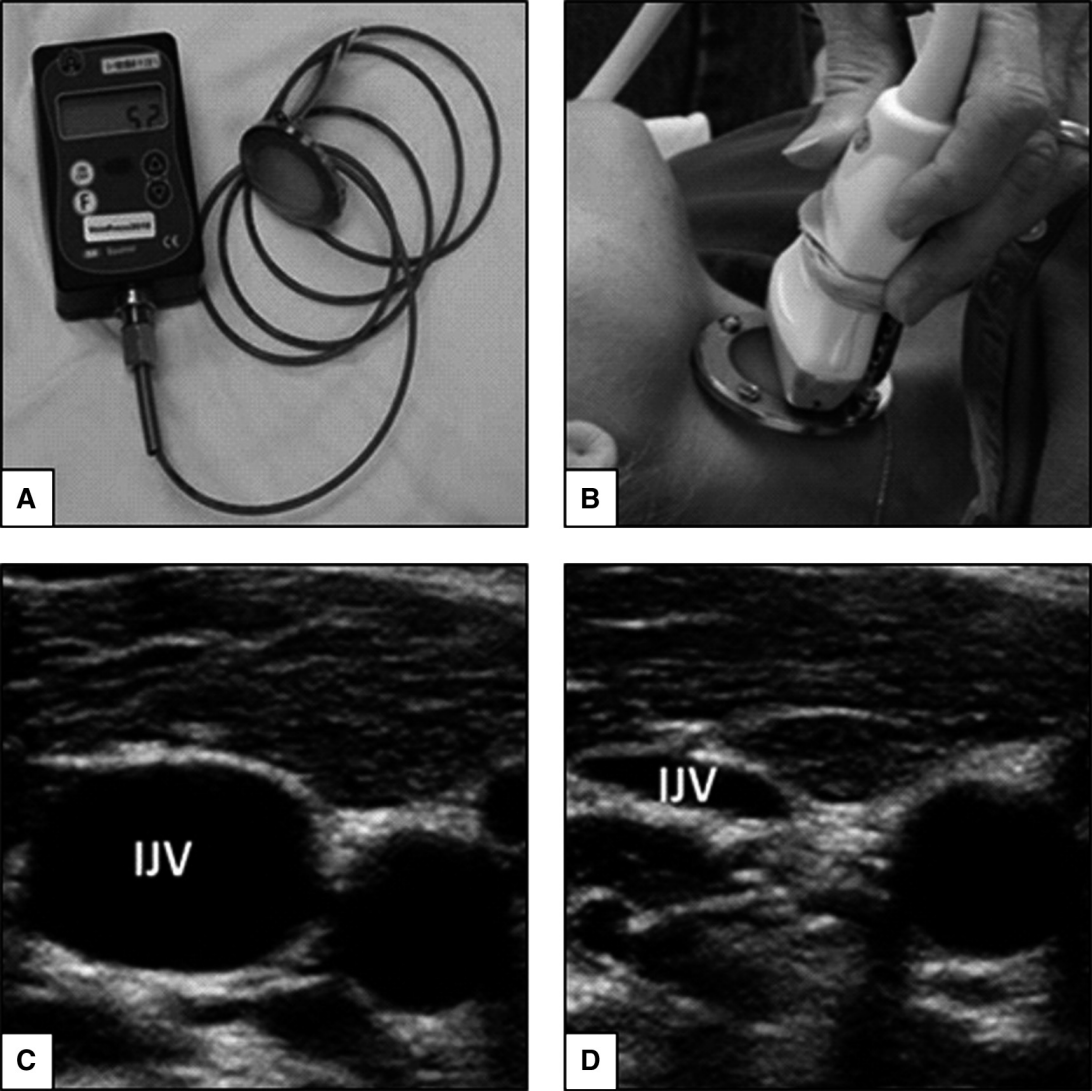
VeinPress device by itself (A) and positioned as it was used on the ultrasound probe during internal jugular venous pressure (IJVP) measurements (B). An image of an uncompressed (C) and mostly compressed (D) vein.
